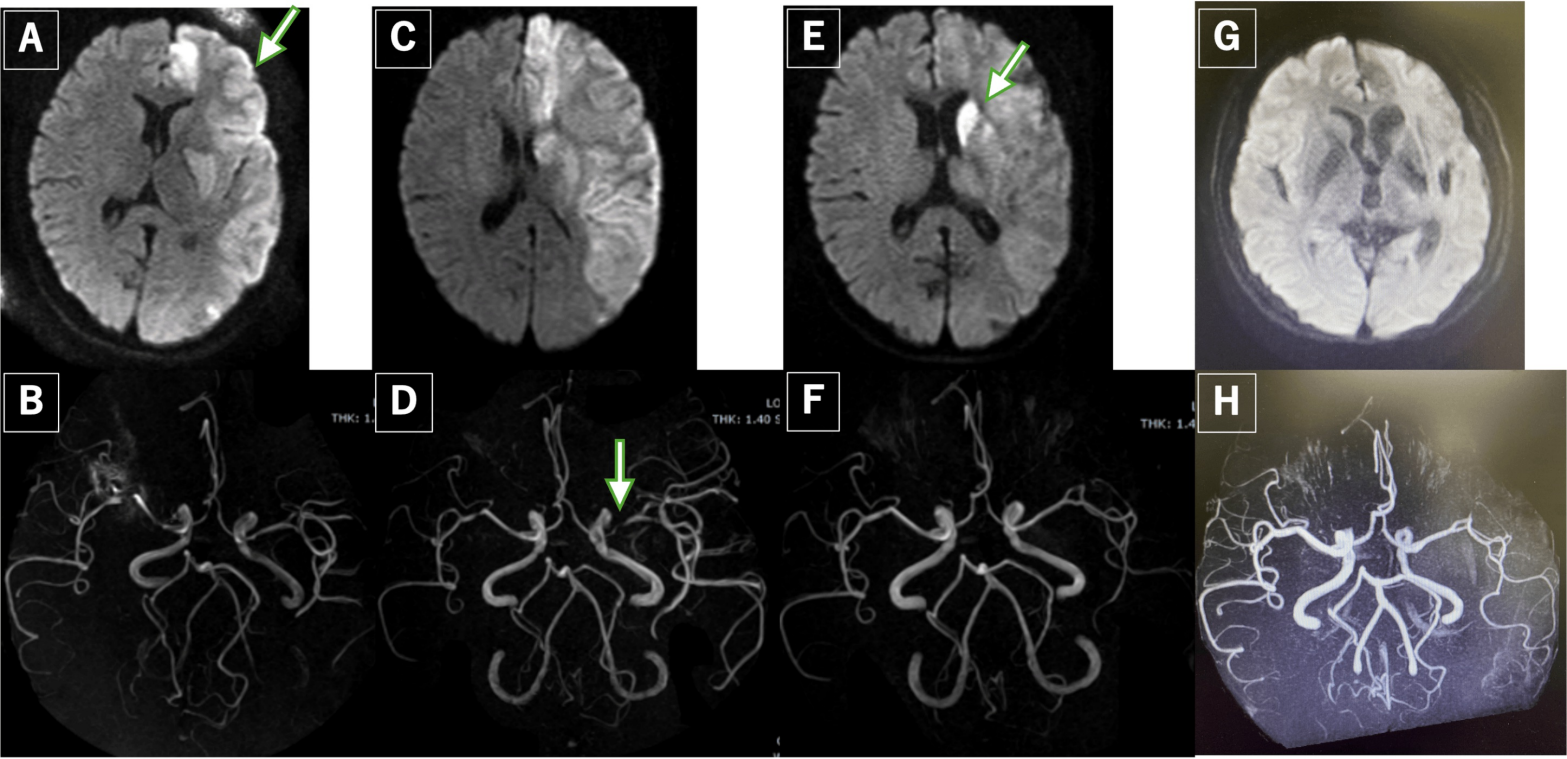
Brain MRI and MRA findings at presentation and follow-up. A: The initial diffusion-weighted imaging (DWI) obtained at the previous institution. The arrow indicates the extensive infarct lesions in the left anterior cerebral artery and middle cerebral artery territories. B: Magnetic resonance angiography (MRA) at the previous institution. C: DWI at our institution indicating the infarct of the left anterior cerebral artery and middle cerebral artery territories. D: MRA at our institution. The arrow indicates the vasospasm. E: DWI one month after initial presentation. New infarct lesions are identified in the left caudate nucleus and putamen (arrow). F: MRA one month after the initial presentation. G: A follow-up MRI performed six months later. H: MRA performed six months later showing resolution of the stenosis in the left anterior cerebral artery and middle cerebral artery.
